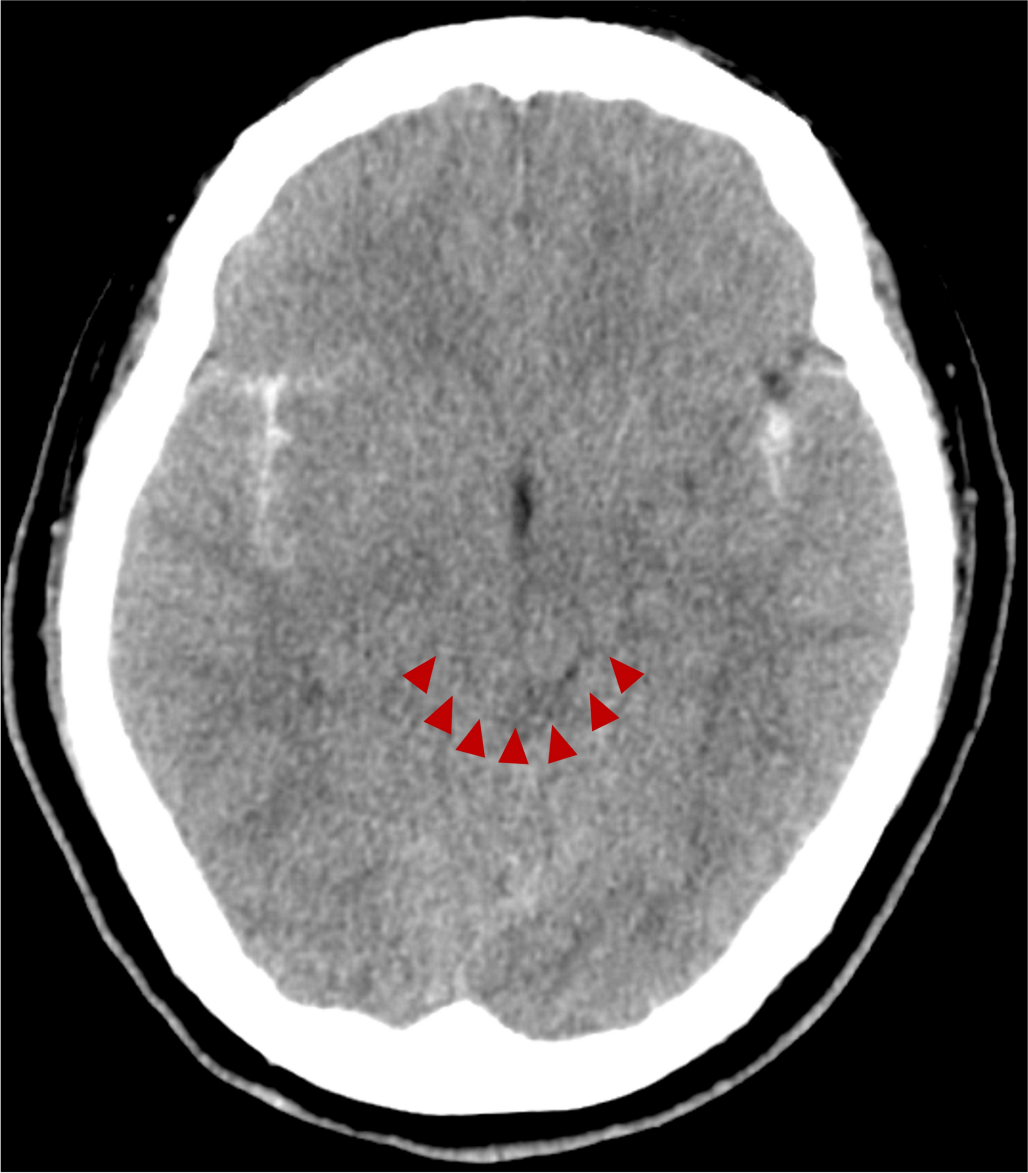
CT Head on postoperative day 1 Head CT showed narrowing of the ambient cistern and quadrigeminal cistern (red arrow head), suggesting increased intracranial pressure, and a lumbar drainage was placed.
